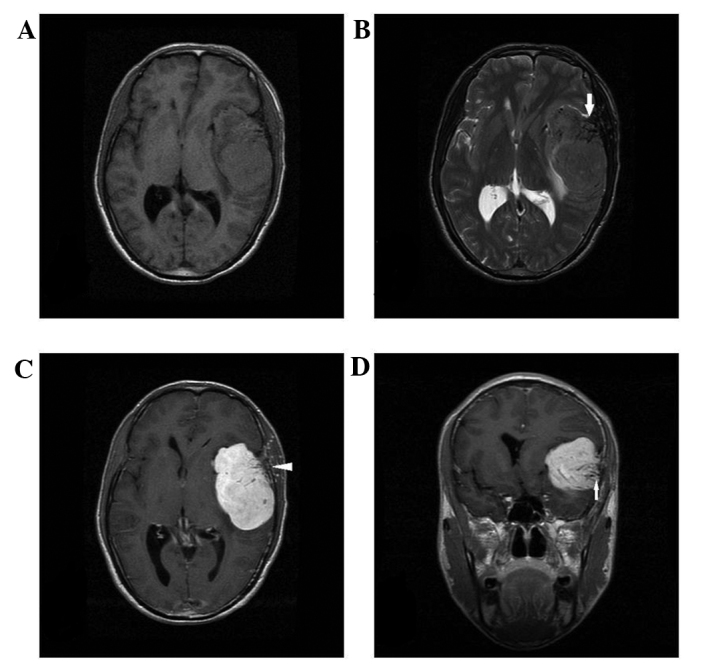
Patient 2. (A) Unenhanced magnetic resonance imaging scan reveals a mass in the left temporal region with heterogeneous iso-signal intensity in the T1-weighted image, and (B) heterogeneous marginally high signal intensity with numerous irregular intratumoral vessels (arrow) in the T2-weighted image. (C) Enhanced scan reveals that the mass is markedly enhanced. The adjacent bone exerts an obstruction effect due to the invasive growth pattern of the tumor (triangle). (D) Coronal reconstruction indicates that the flowing void effect may be visible (arrow).
